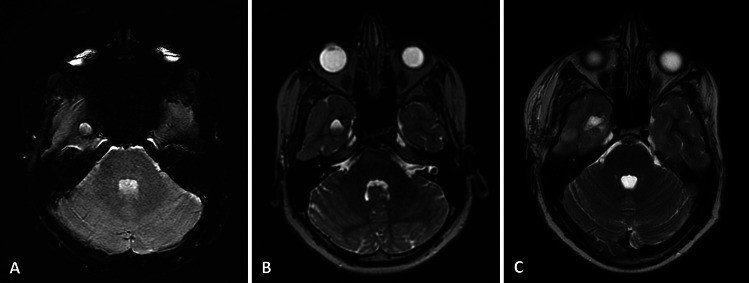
A 12-year-old male asymptomatic patient with an incidentally diagnosed CCM in the right temporal lobe. Preoperative MRI A SWI scan and B T2-weighted scan. The patient underwent gross total removal of the CCM. C Postoperative MRI T2-weighted scan confirmed gross total resection of the lesion
